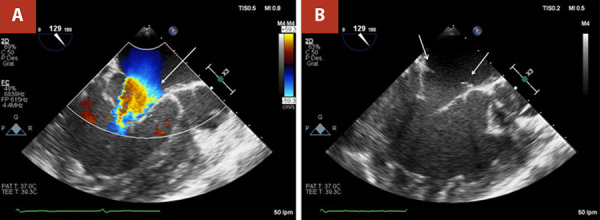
Two-dimensional preoperative transesophageal echocardiography (2D-TEE) with and without color Doppler. (A) 2D-TEE three-chamber view showing turbulent flow (white arrow) and (B) Supravalvular stenosing ring (white arrows) in the systemic atrioventricular valve at 87 degrees.
